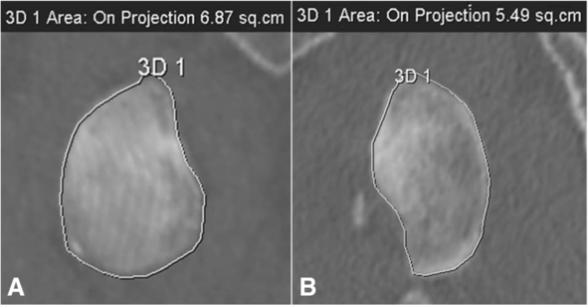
Glenoid bone loss calculated with De Filippo method using CT curved MPR. Normal right glena (a; 6.87 sq. cm), left glena with deficiency (b; 5.49 sq. cm)
